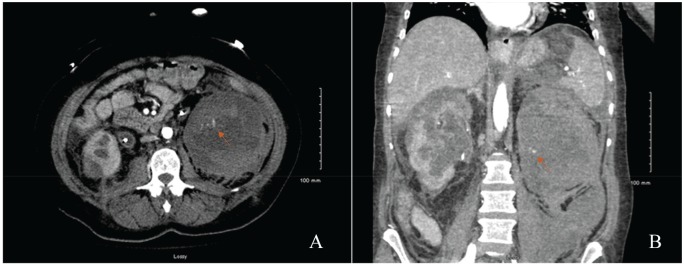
Triple-phase computed tomography of abdomen (A) and pelvis (B) demonstrates progression of the left-sided subcapsular hematoma measuring 10.7 × 10.7 ×16.8 cm with visualization of arterial extravasation on the left kidney (orange arrows).
